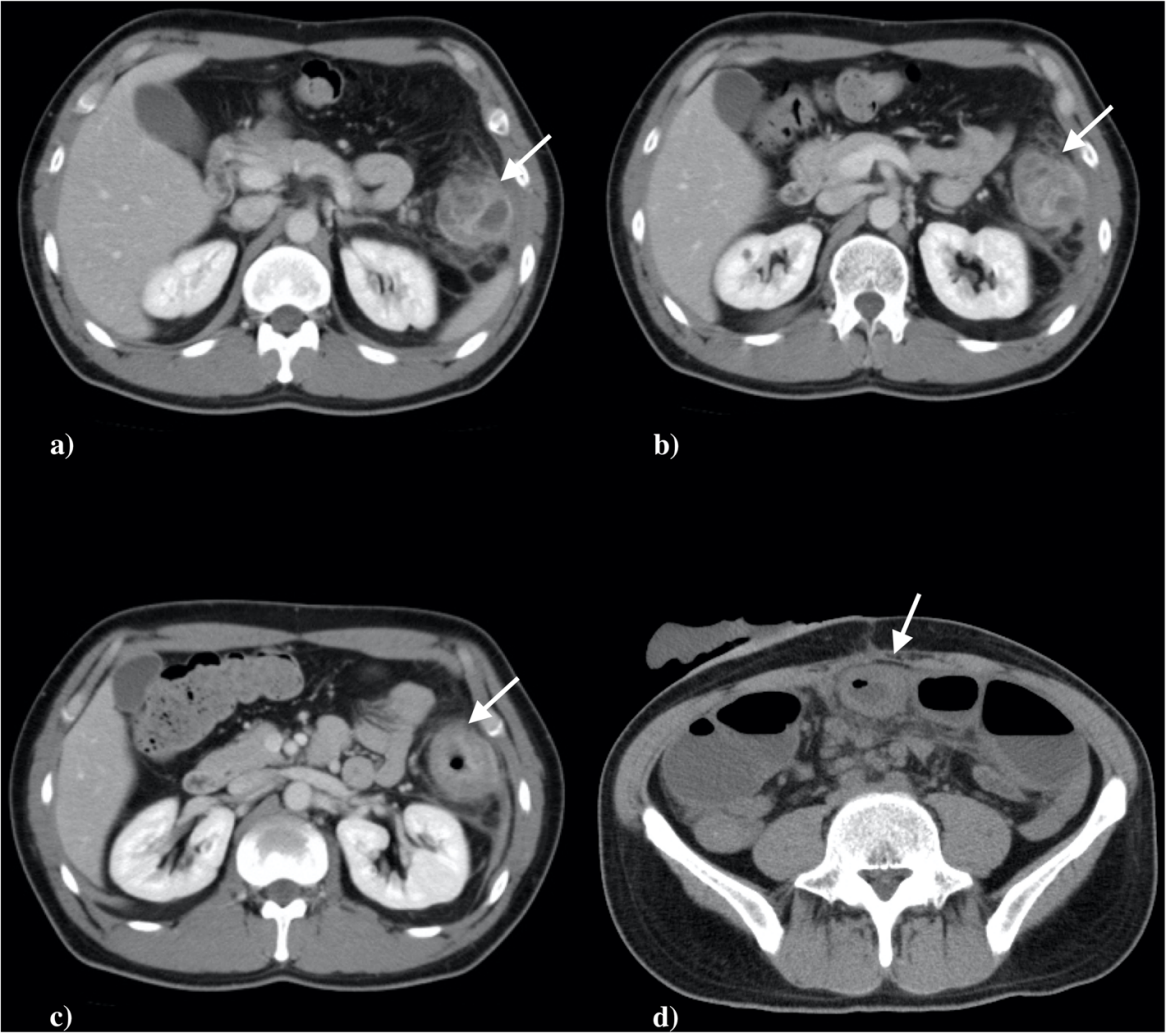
a–d Enhanced CT scan of abdomen showed highly enhanced thickened wall of the colon in the splenic flexure (arrow)
